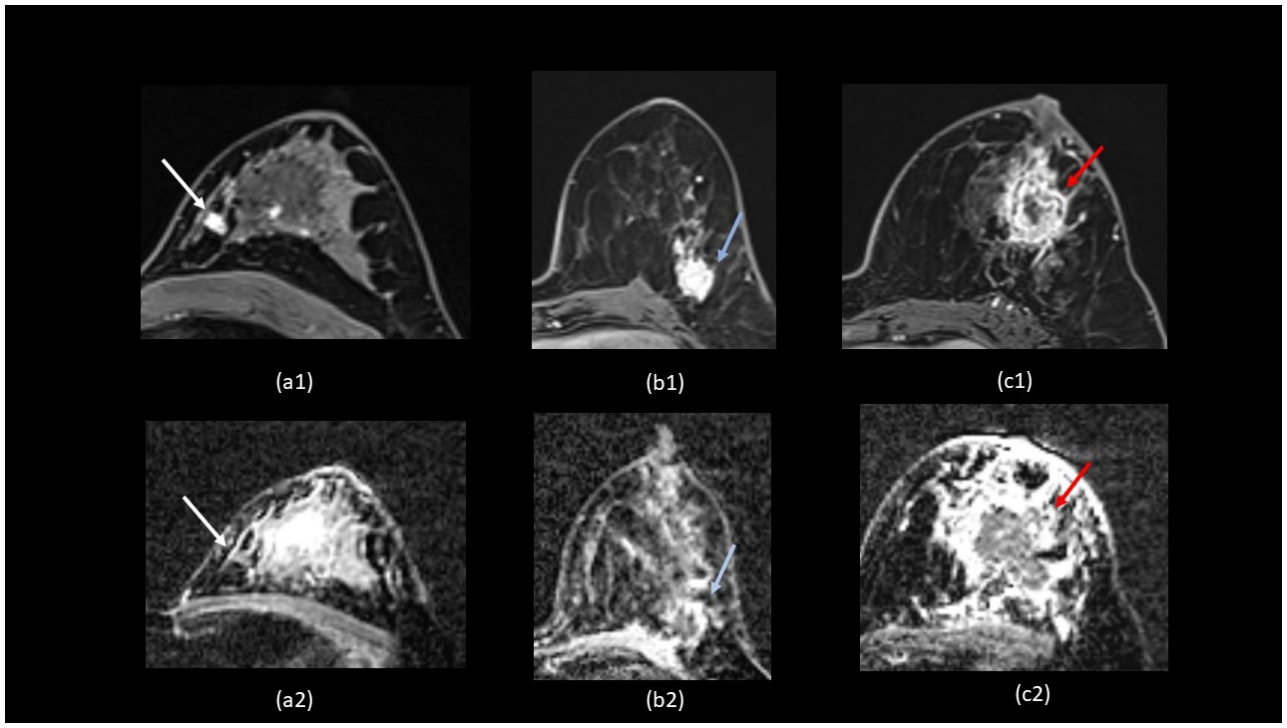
MRI T1-weighted post-contrast (1) and ADC image (2) of each breast cancer subtype. (a) Luminal-like breast cancer (ER- and PR-positive). The lesion (white arrow) at the left mid-inner region is irregular and homogeneously enhanced (a1). The ADC has a low signal intensity with a value of 0.70 x 10-3mm^2^/s (a2). (b) HER-2 enriched subtype, grade 3. The lesion (blue arrow) is irregular with heterogeneous enhancement (b1). The ADC value is 0.91 x 10^-3^mm^2^/s (b2). (c) Triple-negative subtype, grade 3. The lesion (red arrow) is irregular with rim-enhancement (c1). The ADC value is 0.78 x 10^-3^mm^2^/s (c2).
